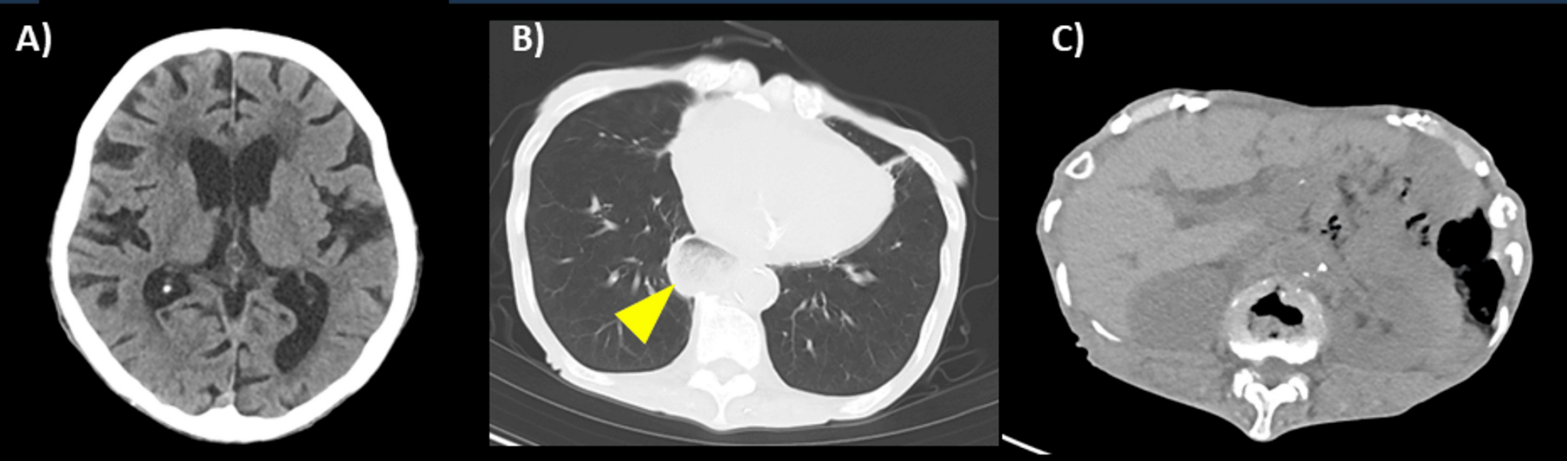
Non-contrast computed tomography on admission. (A) No evidence of intracranial hemorrhage or space-occupying lesion.
